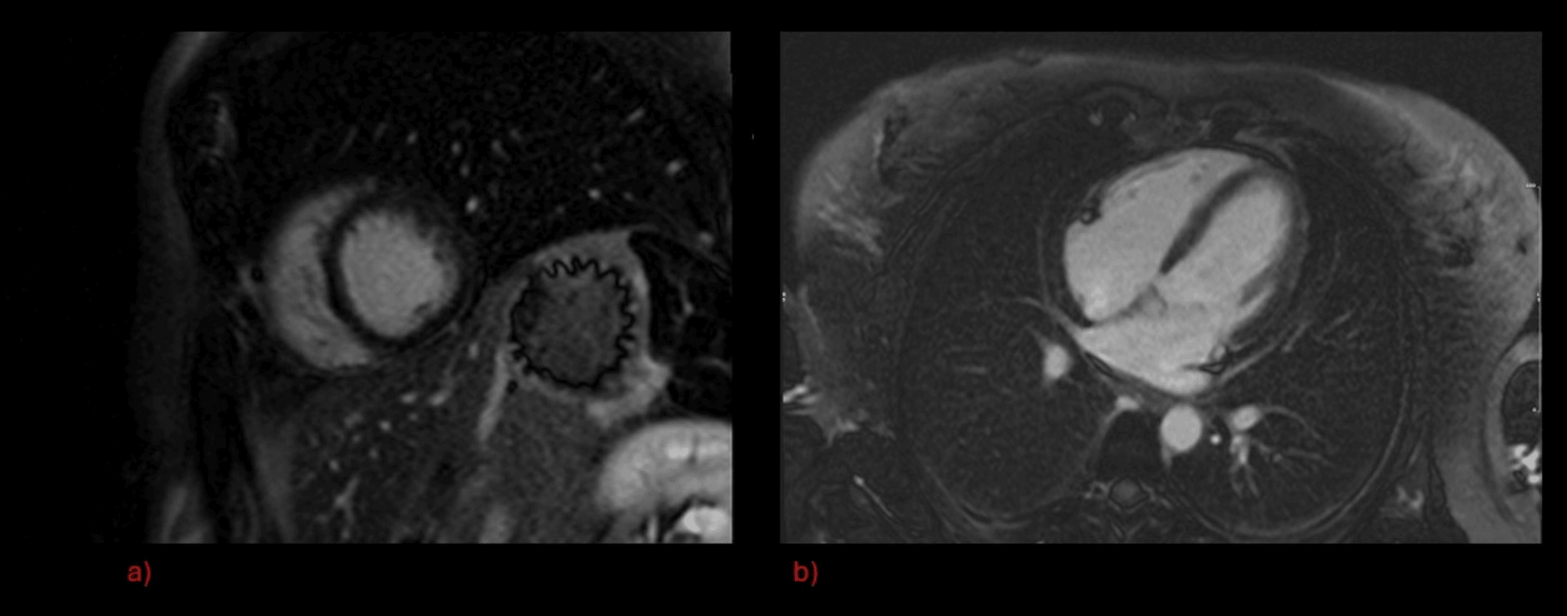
Gadolinium enhanced cardiac MRI during first in-patient episode
